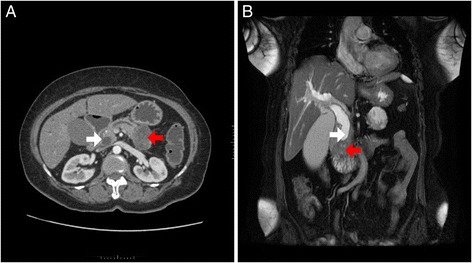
Imaging of the tumours. a – Axial cut of MDCT showing the two tumors of the pancreas. The ampullary tumour (17×19 mm) with ill-defined margins invading adjacent pancreatic tissue (*white arrow*) and the tumor in the tail of the pancreas (28×38 mm) with irregular margins (*red arrow*). b – A coronal MRI image showing the dilated CBD with smooth tapering stricture at the distal end (*white arrow*) and the ampullary tumour inferior to the distal end of CBD (*red arrow*)
